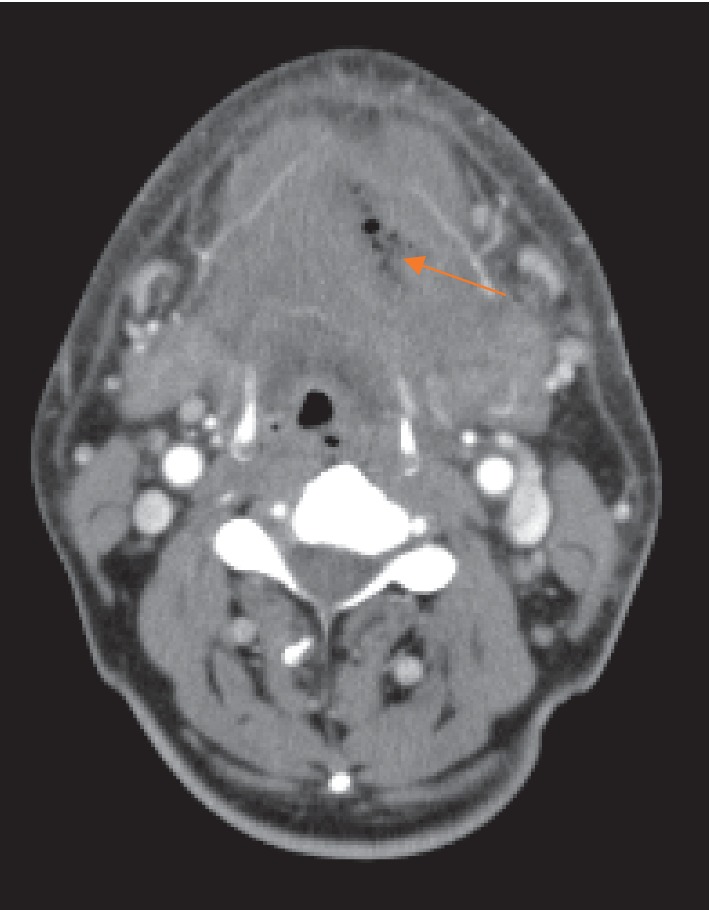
Typical CT image in the first examination. Gas was noted in the left submandibular region over the submental region.
